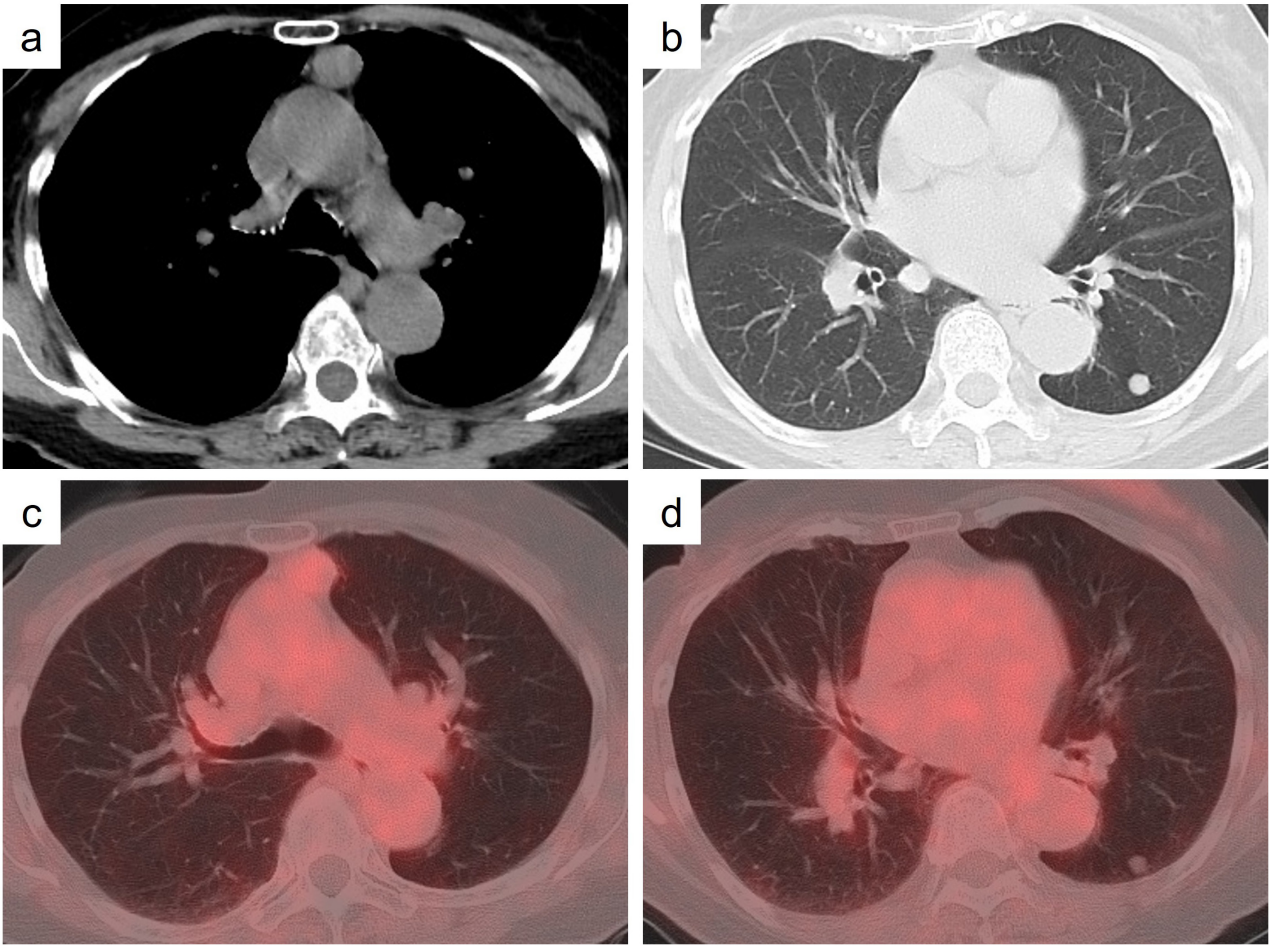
Radiological findings. Chest computed tomography shows (a) a well‐defined nodule in the anterior mediastinum and (b) a solid nodule in the left lower lobe. Positron emission tomography reveals (c) slight accumulation on the anterior mediastinal nodule and (d) no accumulation on the nodule in the left lower lung.
